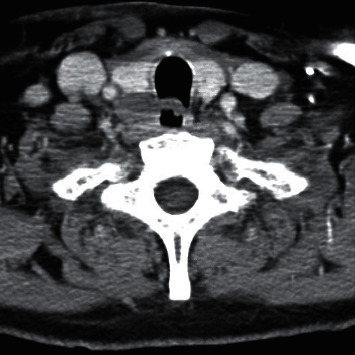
CT scan of the neck after 25 doses of pembrolizumab (axial image).
